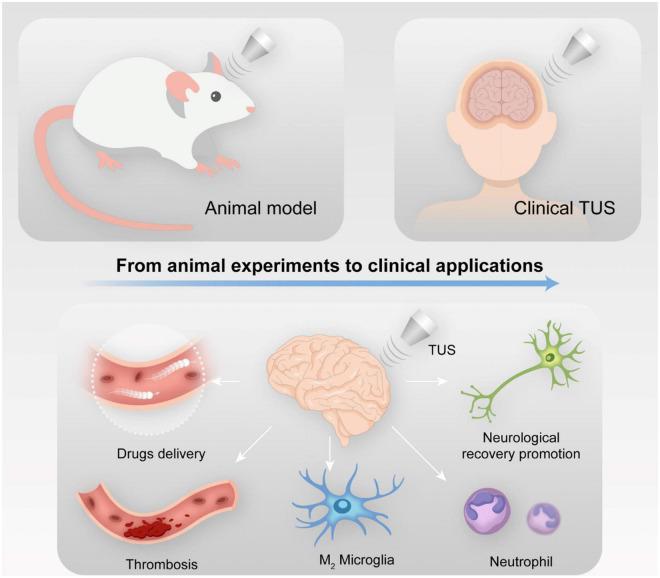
The mechanism of transcranial ultrasound stimulation (TUS) in stroke rehabilitation.
